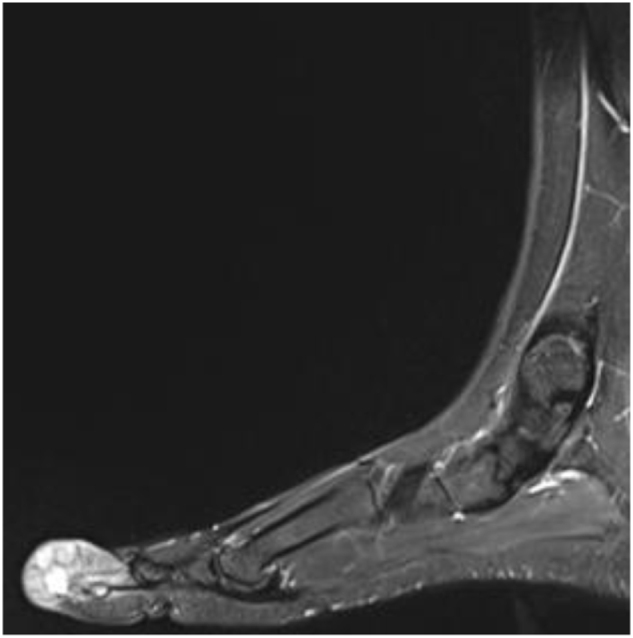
Axial T1-weighted MRI of the left hallux showing a low-signal lesion in the distal phalanx with an associated soft-tissue component.
